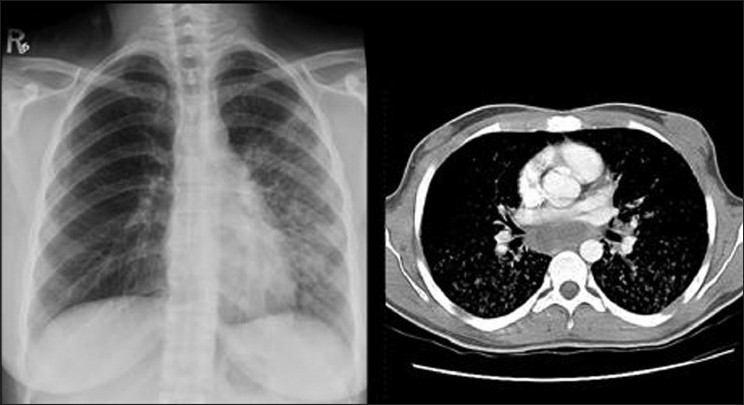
A CXR of lung basis of 47-year-old woman with HPS shows basilar reticulonodular opacities at the left lung base and left mid-zone. These opacities which represent dilated lung vessels are seen on both sides on CT as shown
